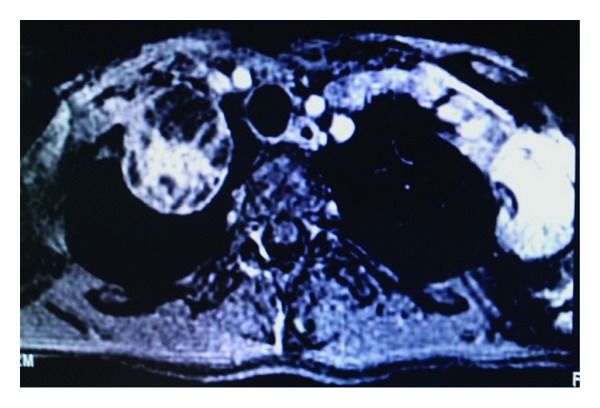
Axial T1 weighted fat saturated postcontrast MR image showing two large extraspinal upper thoracic heterogeneous extraspinal neurofibromas.
